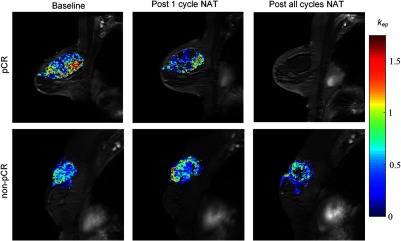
An example of quantitative analysis of DCE-MRI data of a patient achieving pCR (top row) and a patient not achieving pCR (bottom row) before (first column), after the first cycle (second column), and at conclusion of all NAT (third column). The parametric map of $k_{\mathrm{ep}}$ overlaid on a high resolution anatomical MRI scan is shown. Note the diminution of $k_{\mathrm{ep}}$ exhibited in the patient achieving pCR after one cycle of NAT, but the enhanced $k_{\mathrm{ep}}$ in the patient not achieving pCR.
